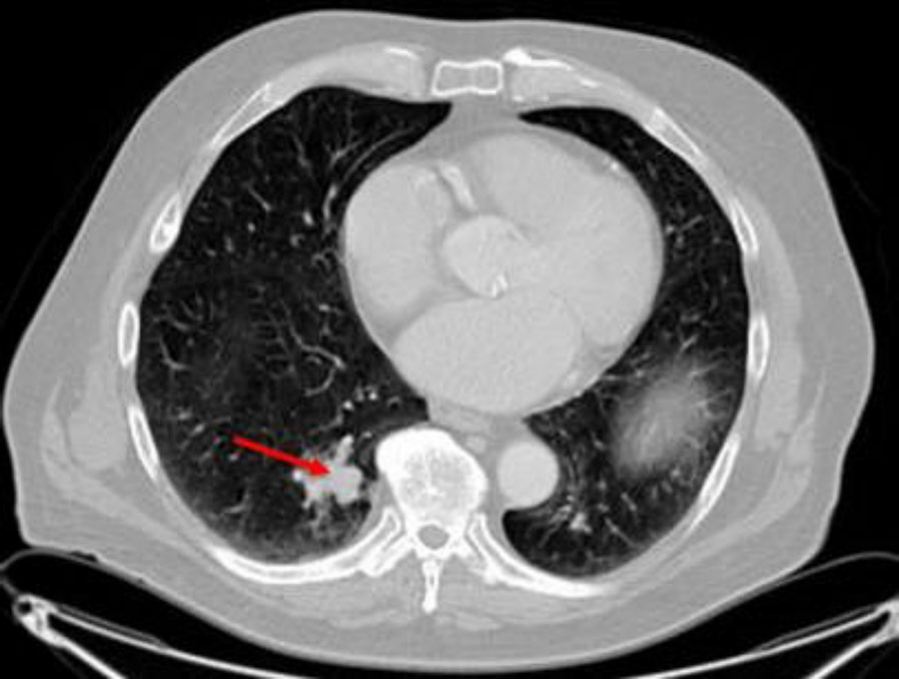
CT scan of chest. Red arrow indicates lung metastasis. PET scan and CT chest, abdomen and pelvis were only positive for the isolated lung metastasis
